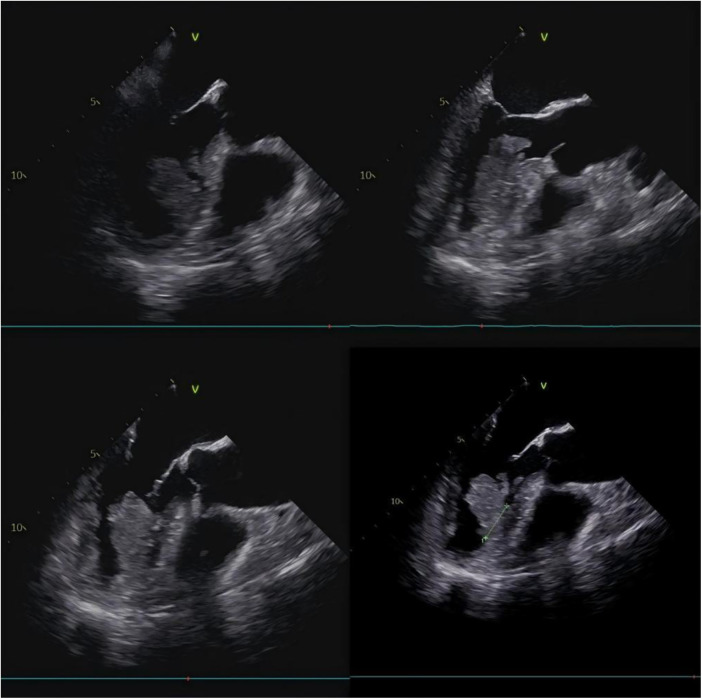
Preoperative transesophageal echocardiography demonstrating left ventricular cavity with multi-angle assessment of mass morphology, dimensions, and basal attachment.
